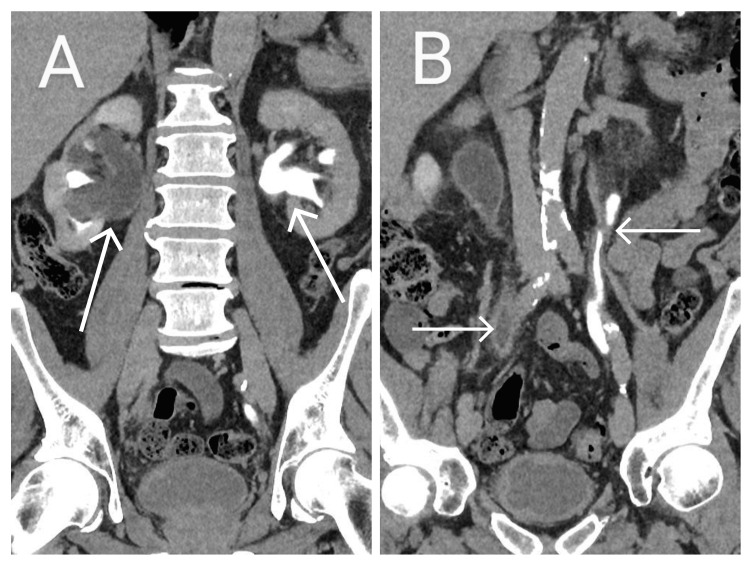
Computed tomography urogram showing hydronephrosis (arrow) (A) and wall thickening and filling defect in both ureters (arrow) (B).
